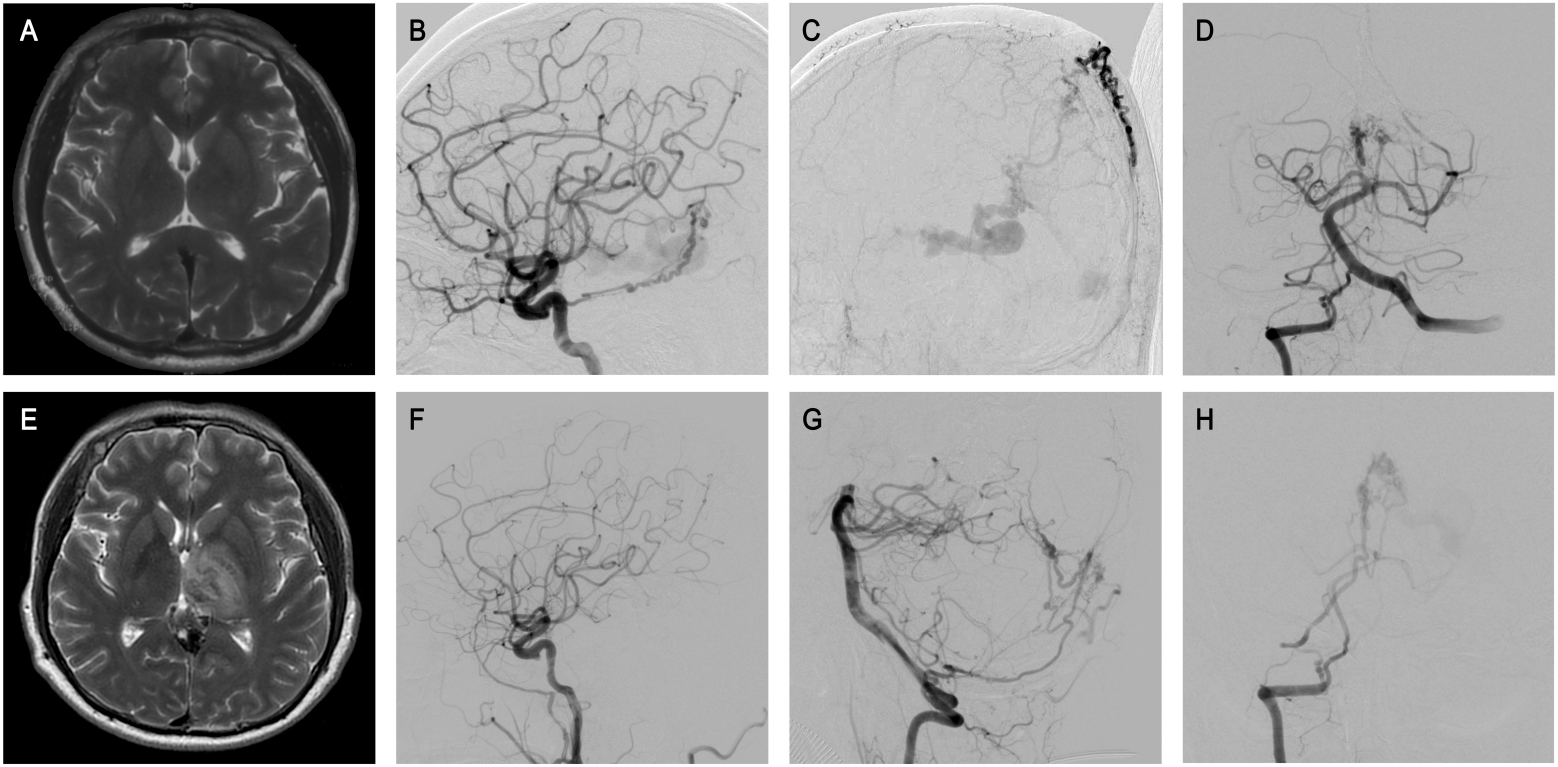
The patient 33 presented that physical examination revealed intracranial vascular malformation for 3 months. (A) Preoperative MRI showed no obvious abnormalities. (B–D) ICA and VA, and ECA angiography revealed GDAVF feeded by the bilateral MMAs, OAs, MHTs, and right PMA, left IMA, left MPChA. (E) Half a month later, MRI sacan showed abnormal signals in bilateral thalamus area in T2, which was the manifestation of venous infarction. (F–H) Postoperative angiographic image. Postoperative angiogram images showing complete obliteration of the GDAVF (F, G). Right VA arteriography suggested the intravenous outflow time of contrast agent was delayed (H).
